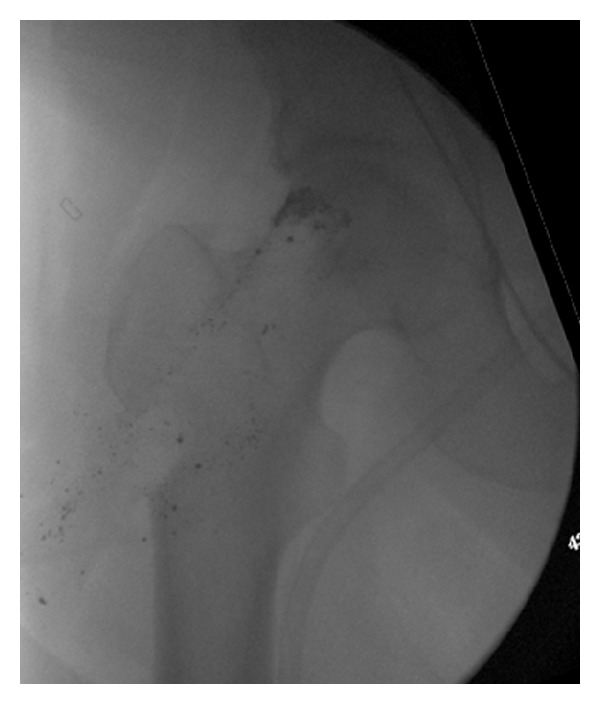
Postoperative radiograph of right proximal femur.
